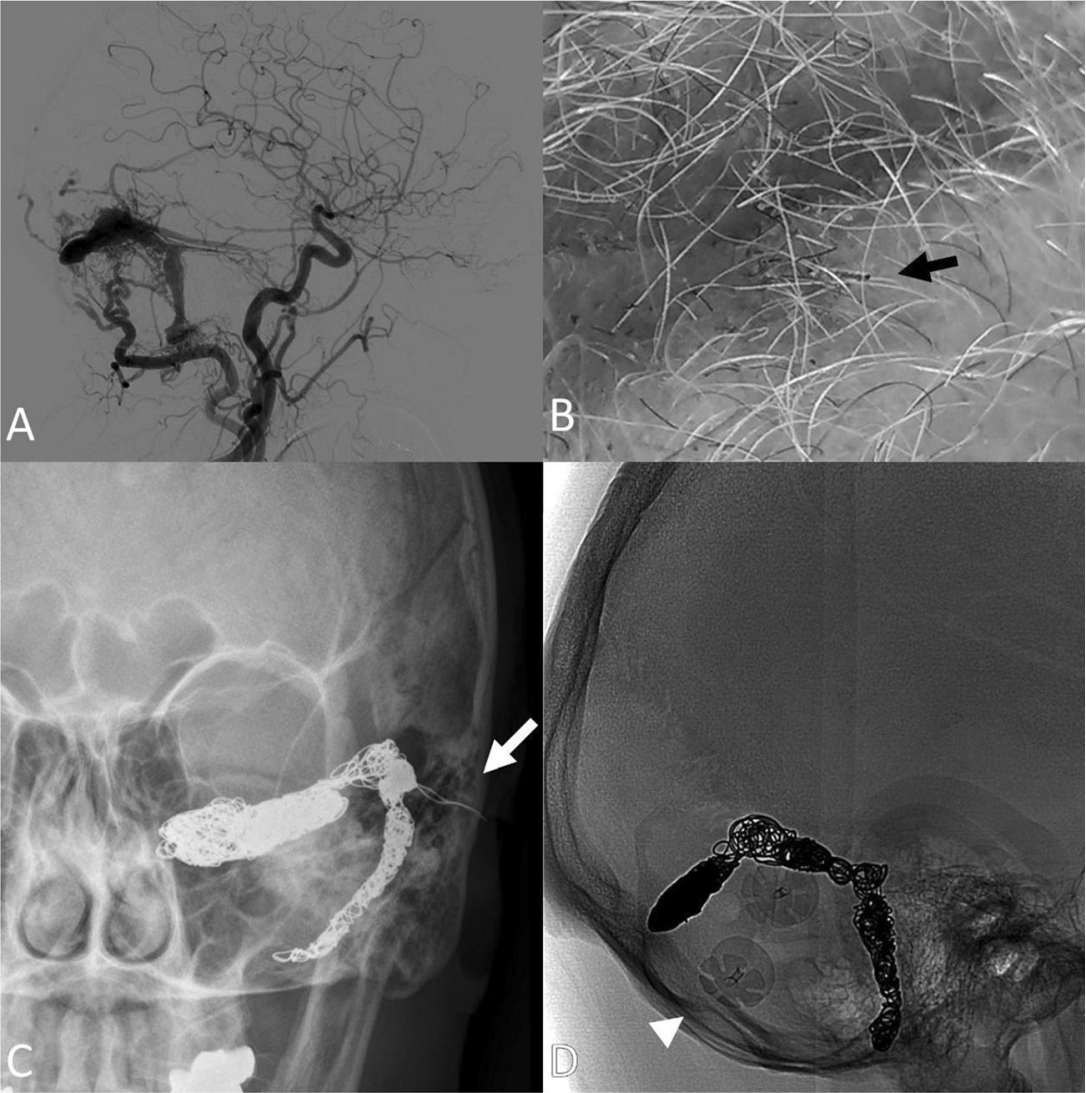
a Lateral projection digital subtraction angiography demonstrating the arteriovenous fistula with shunting from the left occipital artery and left medial tentorial artery, and with early cortical reflux. b Photograph demonstrating the protruding coil (black arrow). c Anterior-posterior projection x-ray of the skull demonstrating the coil embolization mass with protrusion of the coil extracranially through the craniectomy defect (white arrow). d Lateral projection native fluoroscopic image after craniotomy for resection of migrated coil (absent coil strand along the embolization-access tract) with reconstructive plates (arrowhead)
